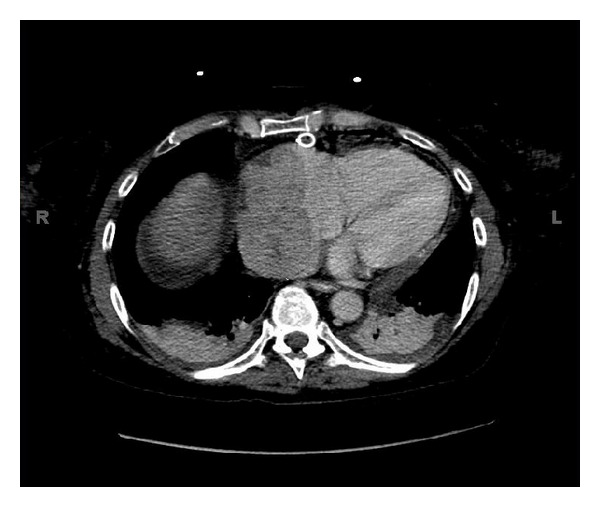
Right cardiac atrial mass shown by CT scan extending into the inferior vena cava, involving the superior vena cava as it enters the atrium, and pericardial effusion.
